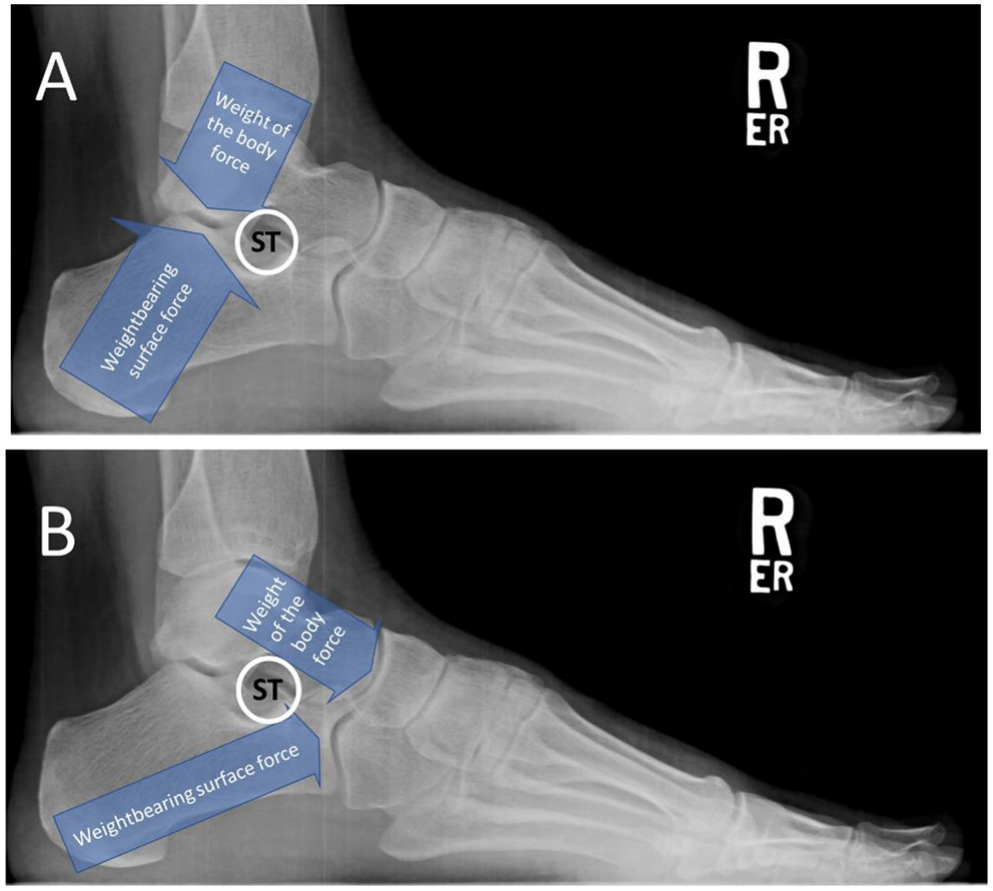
Weightbearing lateral foot radiograph of a foot in resting stance position showing normal talotarsal joint forces. (A) Forces acting posterior to the sinus tarsi at heel strike. The sinus tarsi is labeled as ST. (B) Forces acting anterior/distal to the sinus tarsi as the plantar surface of the foot makes contact with the weightbearing surface. Sinus tarsi should remain open.
